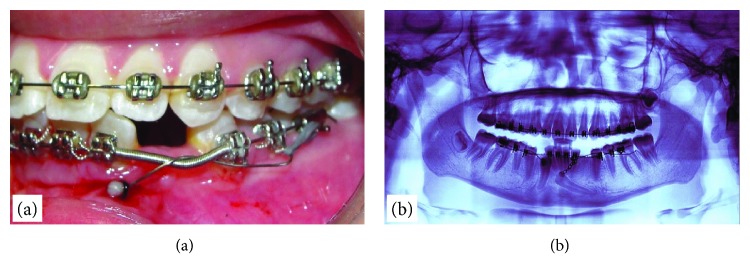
(a) An intraoral photograph of the quadrant 3 accessorial wire tied up to the golden chain. (b) Progress panoramic radiograph.
